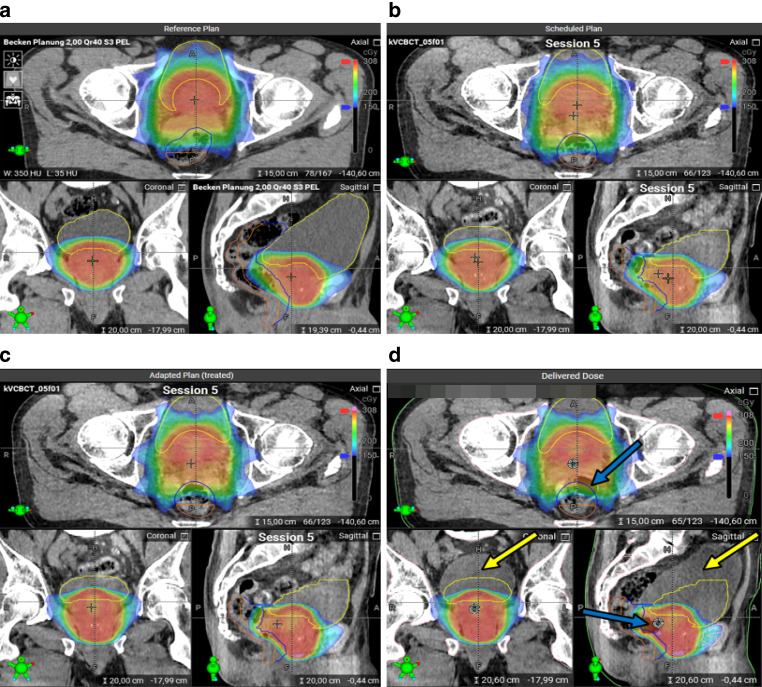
Original and adapted plans on different (CB)CT images for the same treatment session. a The planning CT with the original treatment plan. b The original plan on CBCT1. c The adapted plan on CBCT1. d The adapted plan on CBCT2, with contours taken from CBCT1. As can be seen, particularly the bladder has changed its volume between CBCT1 and CBCT2, and an air pocket has moved down the rectum. Parts of both OARs lie outside of the contours (the yellow arrows point to the bladder part that is outside of the bladder contour, and the blue arrow to the rectum part). Patient 4, session 5. Contour color scheme: bladder—yellow, rectum—blue, posterior rectum wall—orange
